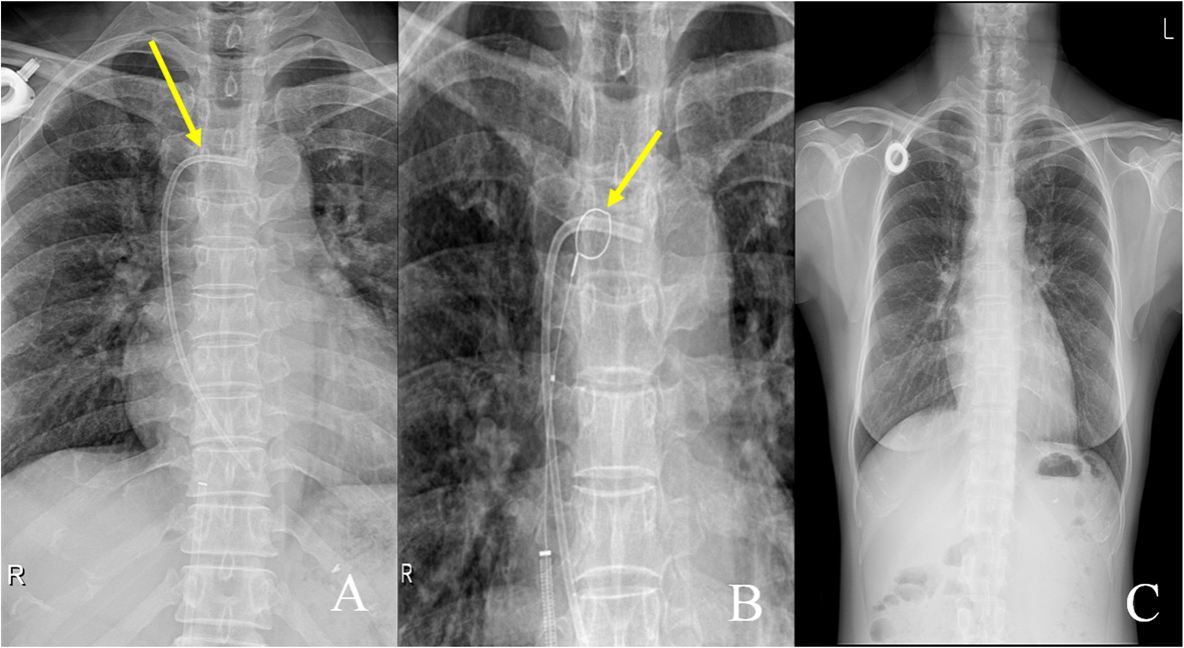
A procedure of removal of fractured catheter of a totally implantable venous access port (TIVAP). a Chest radiography identified the location of a fractured and moved part of a TIVAP. Arrow indicates the fractured catheter fragment. b A fractured and moved part of a TIVAP was caught by a goose neck snare with angiographic guidance. Arrow indicates a goose neck snare. c The fractured and moved catheter was completely removed
